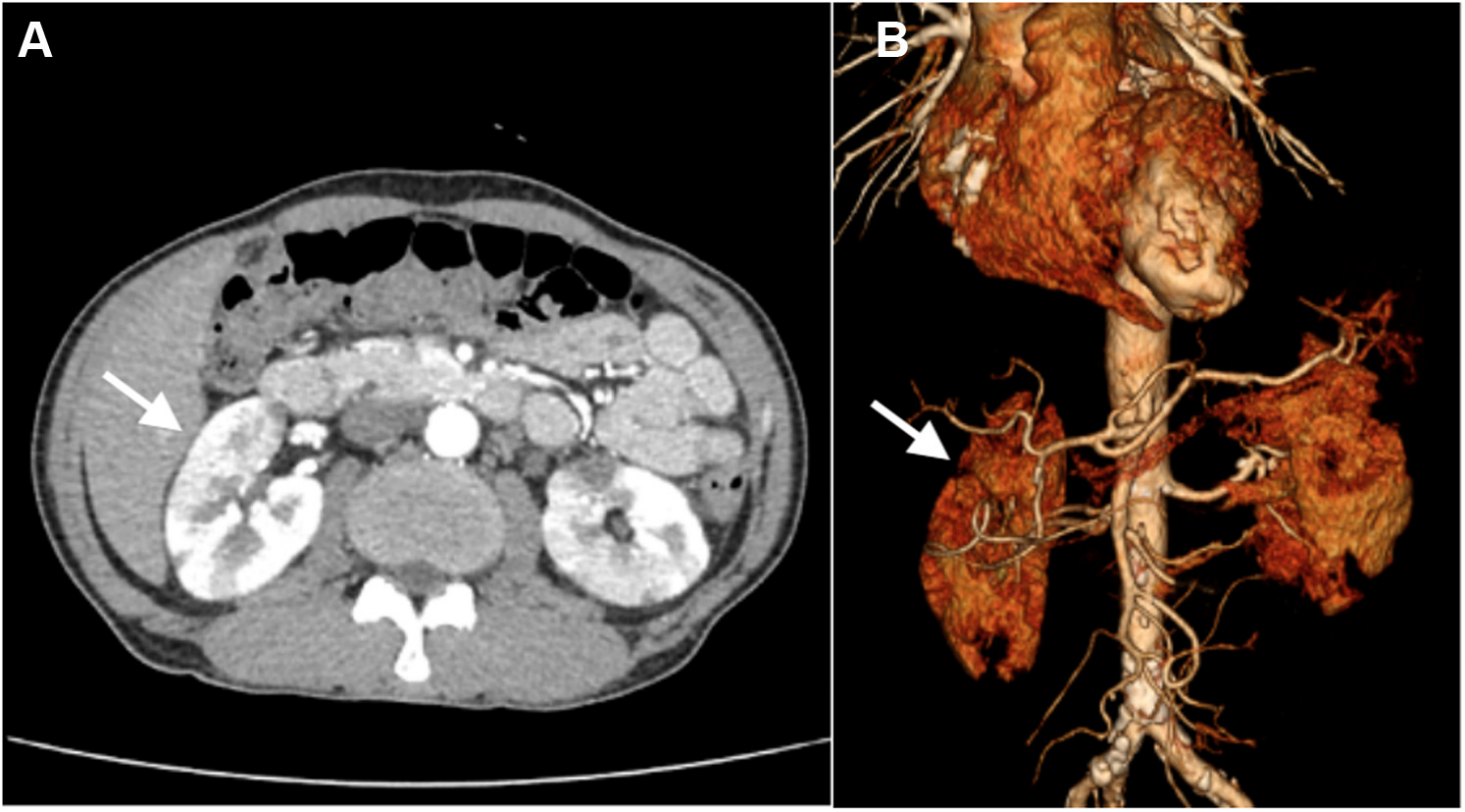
Renal Thrombi Resolution (A and B) Computed tomography demonstratedcomplete resolution of the renal thrombi (arrows).
